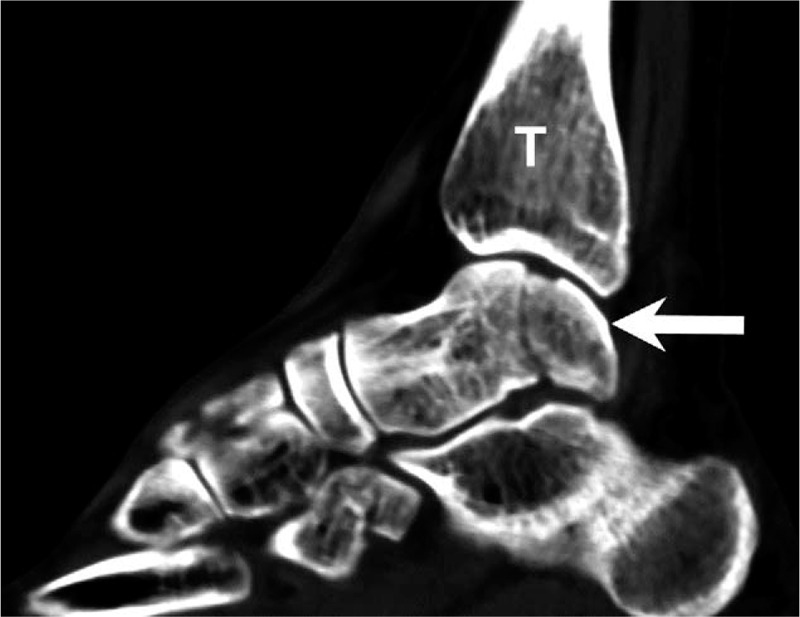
Computed tomography image of the talar body fracture. Arrow = talus, T = tibia.
